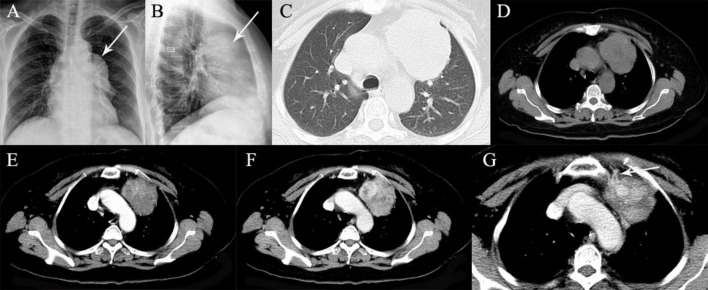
A 60-year-old woman with thymoma. (A,B) Chest radiographs show a well-defined opacity projecting the anterior left hilum (arrows). (C,D) Precontrast CT scans demonstrated a left anterior mediastinal mass with well-delineated margin. (E,F) On biphasic postcontrast images, the mass shows gradual enhancement and heterogeneous marked enhancement in the venous phase. (G) Feeding arteries arising from the left internal thoracic artery are detected (arrow).
